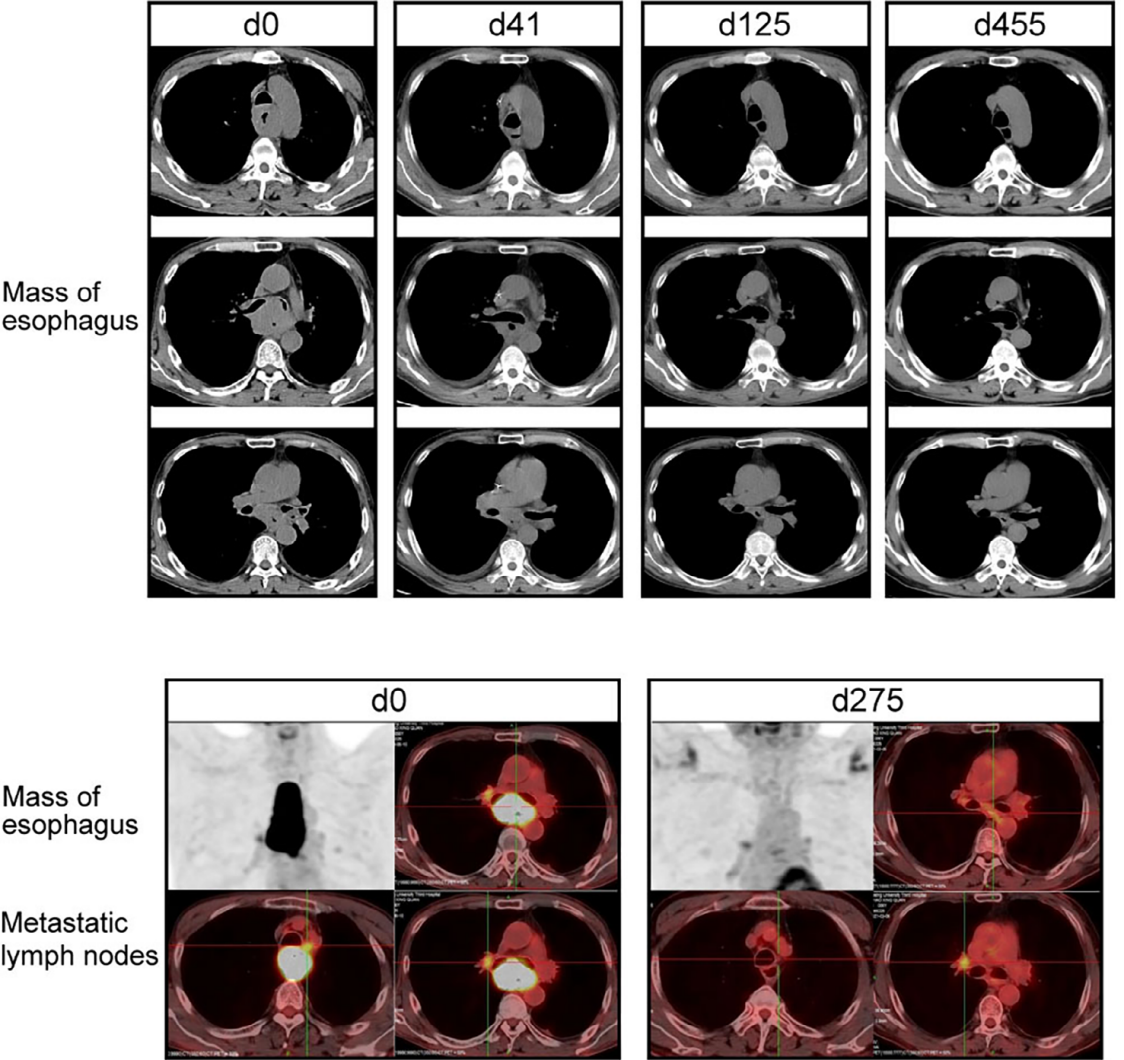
Monitoring tumor response to treatment. Representative computed tomography (CT) and fluorodeoxyglucose (FDG)‐positron emission tomography‐computed tomography (PET‐CT) images of tumor before and after therapy
